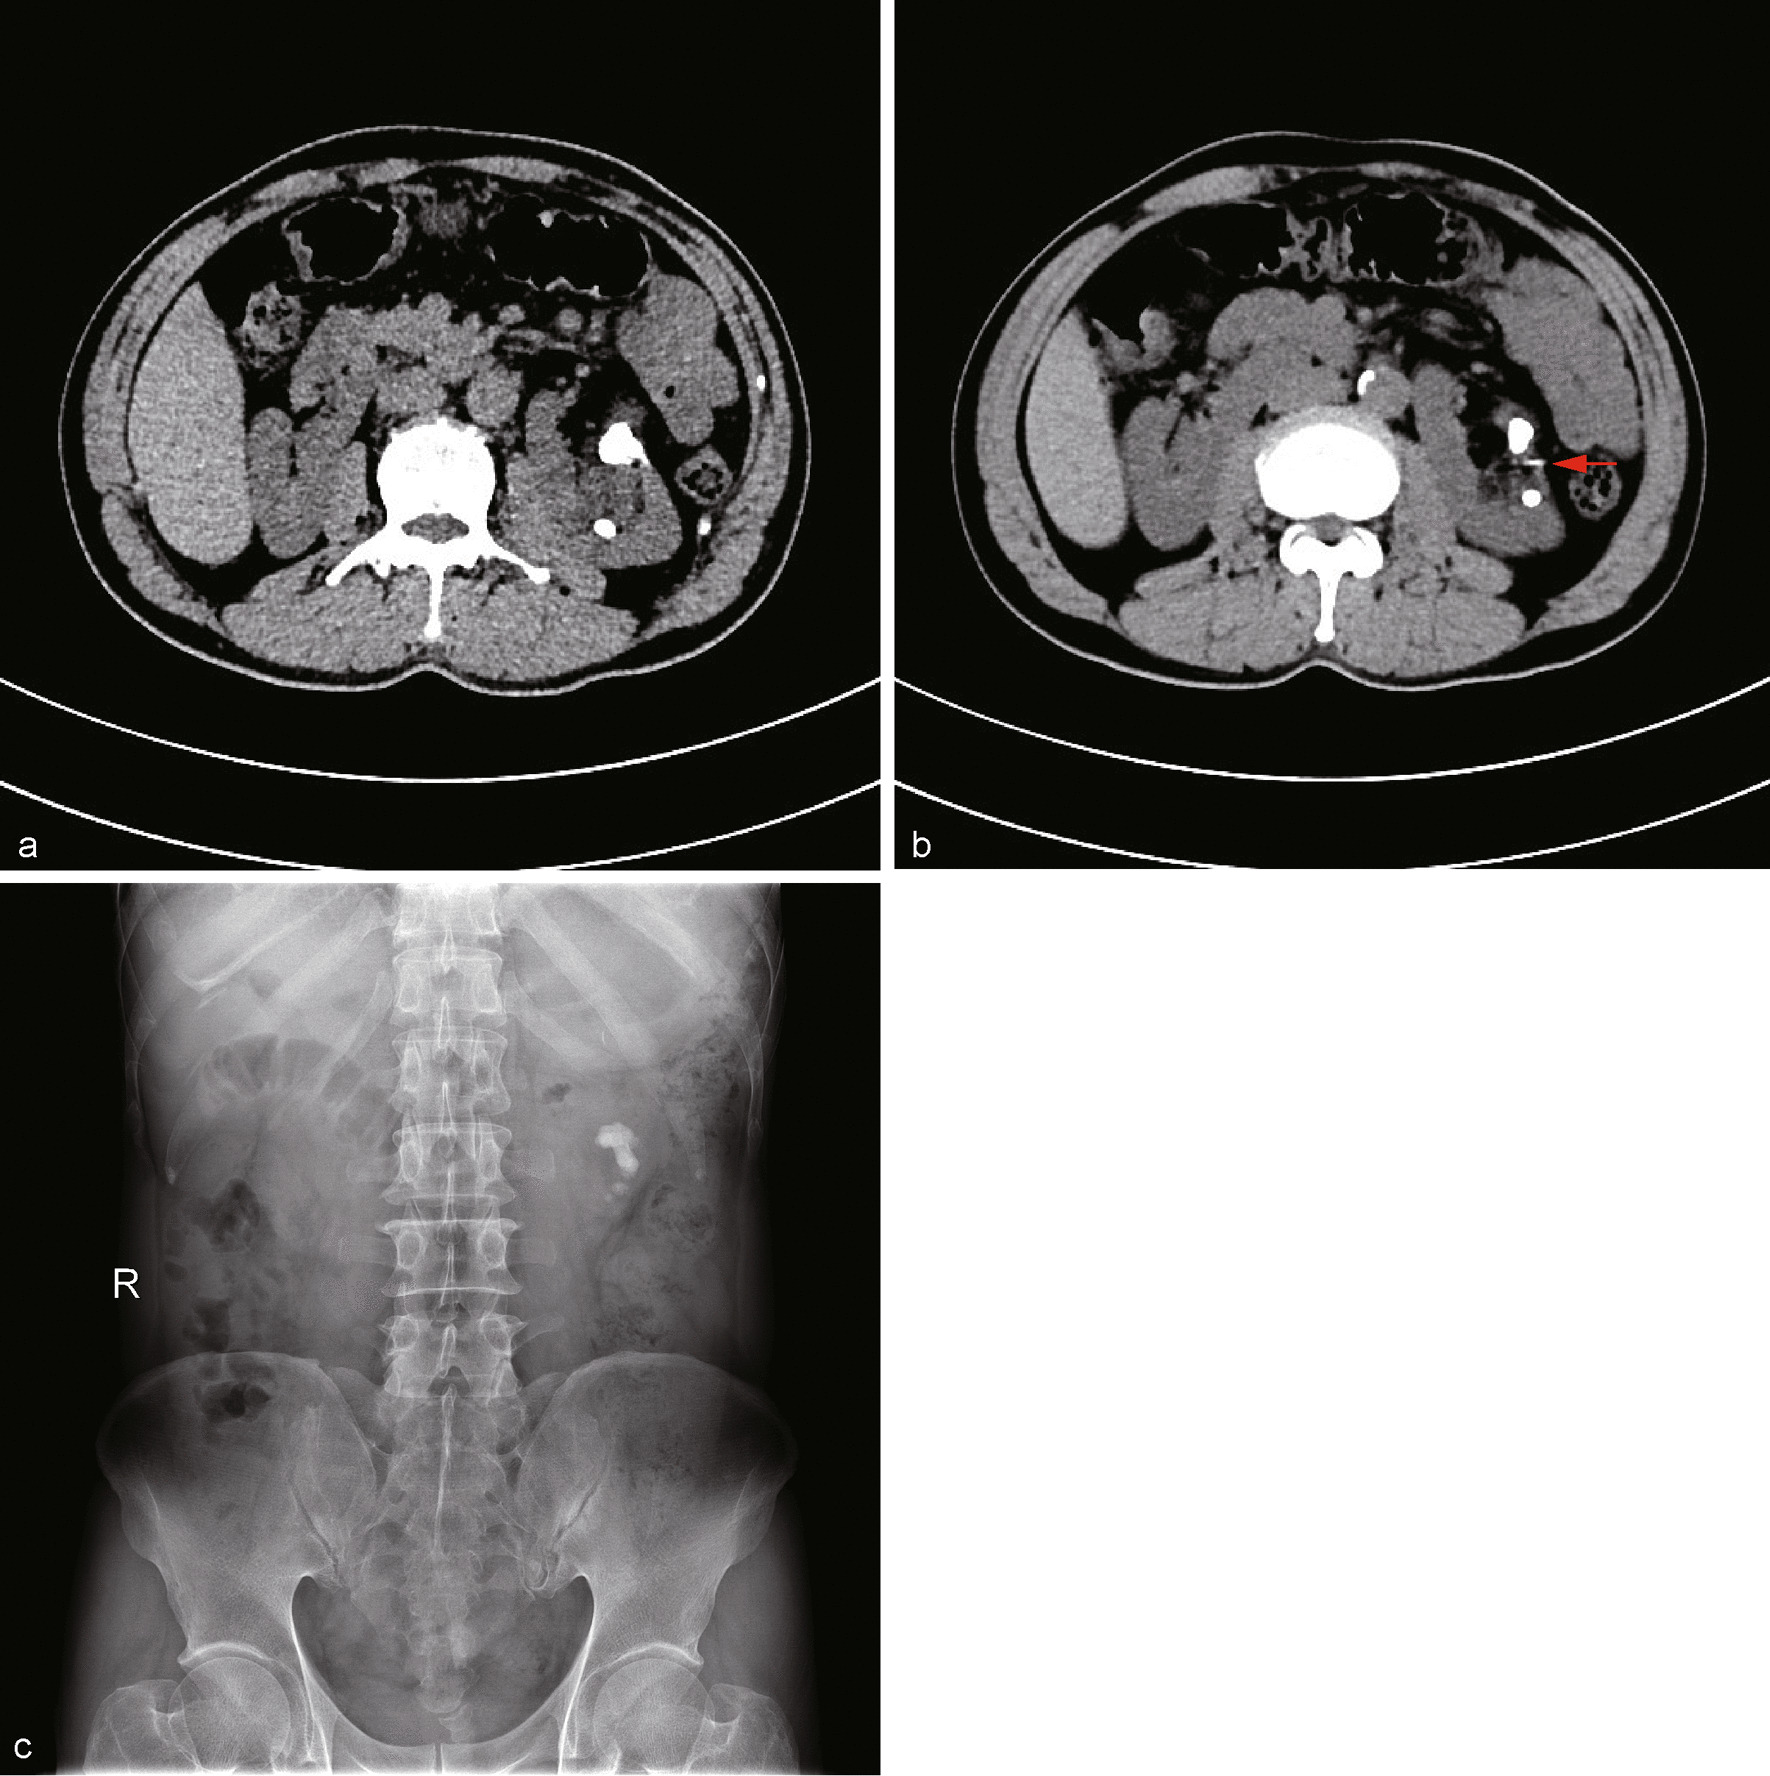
Preoperative Imaging. a CT image showed left renal stones with mild hydronephrosis. b CT scan revealed part of the HOLC imbedded into renal pelvic wall (red arrow). c A KUB plain displayed the left kidney stones about 25 × 20 mm in size
